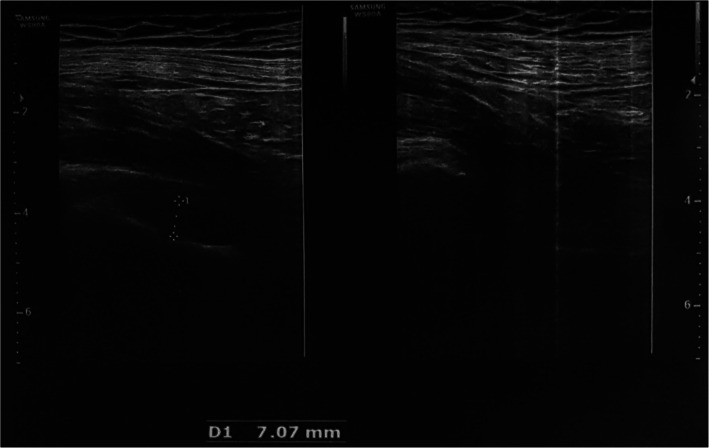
Ultrasonic images of soft tissue around the hip. An increase in thickness is seen in the synovium and also articular effusion in the hip’s neck with a 7 mm diameter
